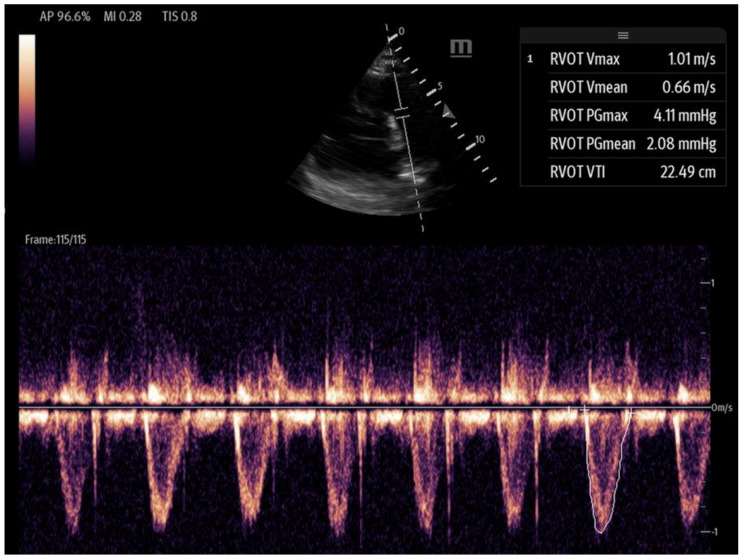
A right ventricular tract VTI in a modified parasternal short axis view, activating the PW doppler.
